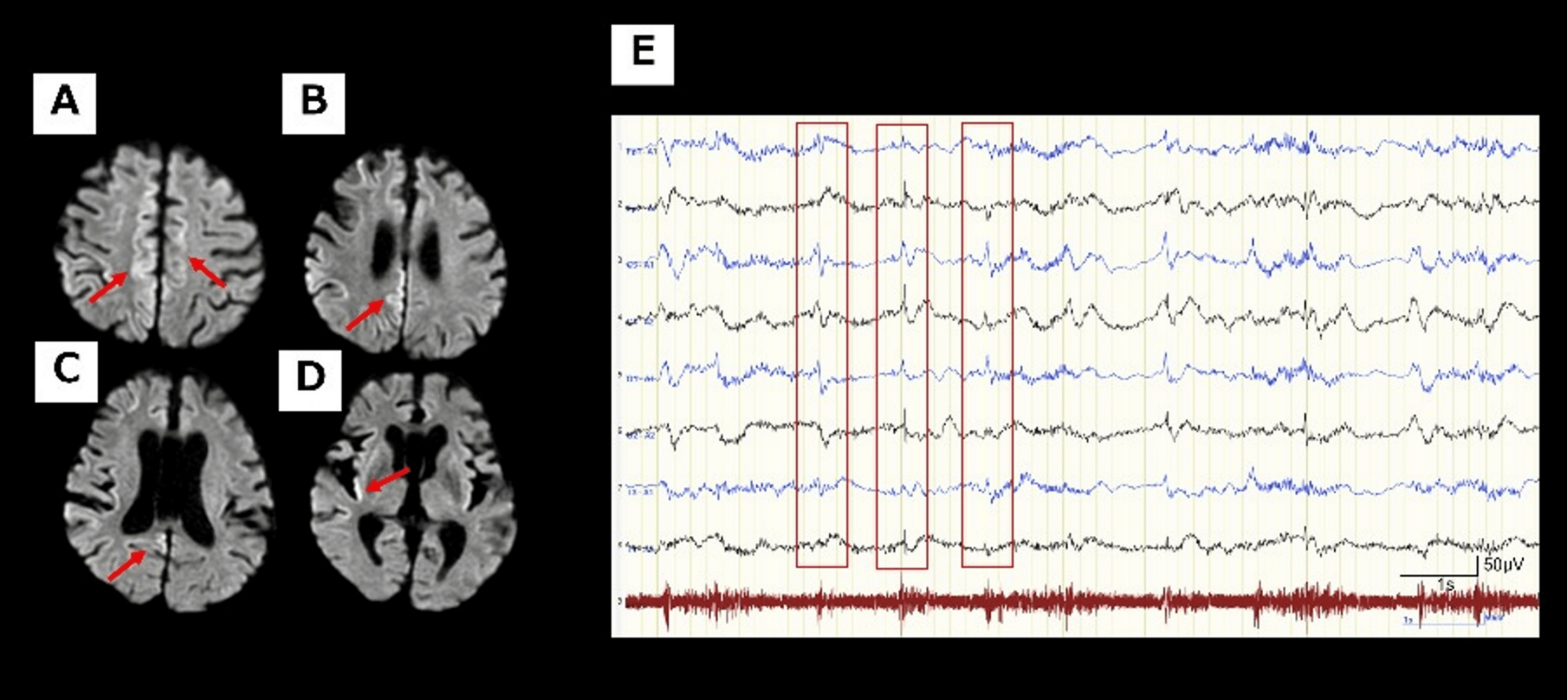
Follow-up MRI and EEG (A-D) Follow-up diffusion-weighted MRI in late August revealed clear hyperintensity in the bilateral parietal cortex (red arrow). EEG in early September revealed periodic sharp wave complexes (PSWCs) at approximately 1 Hz, typical of sCJD. (E) PSWCs were predominantly in the left hemisphere but were almost generalized (red square).
